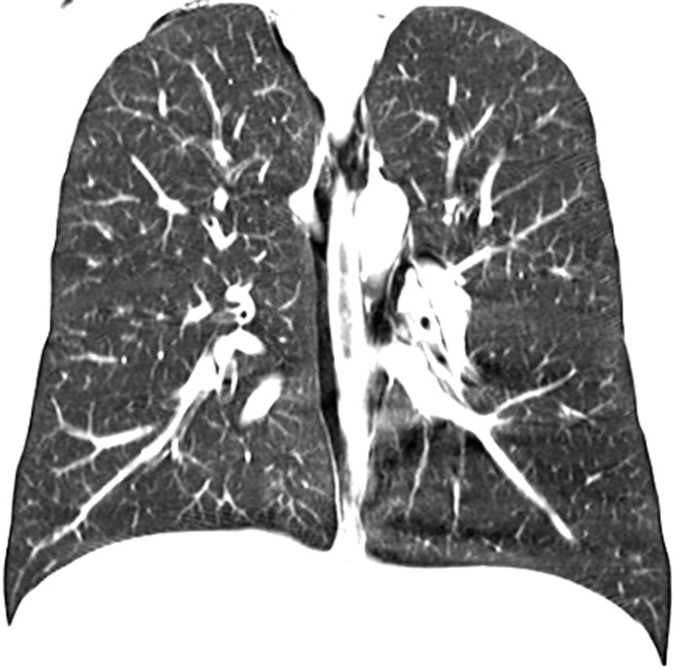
chest CT scan: coronal view showing air pneumomediastinum and discontinuity in the oesophageal wall about 2cm from the gastroesophageal junction
